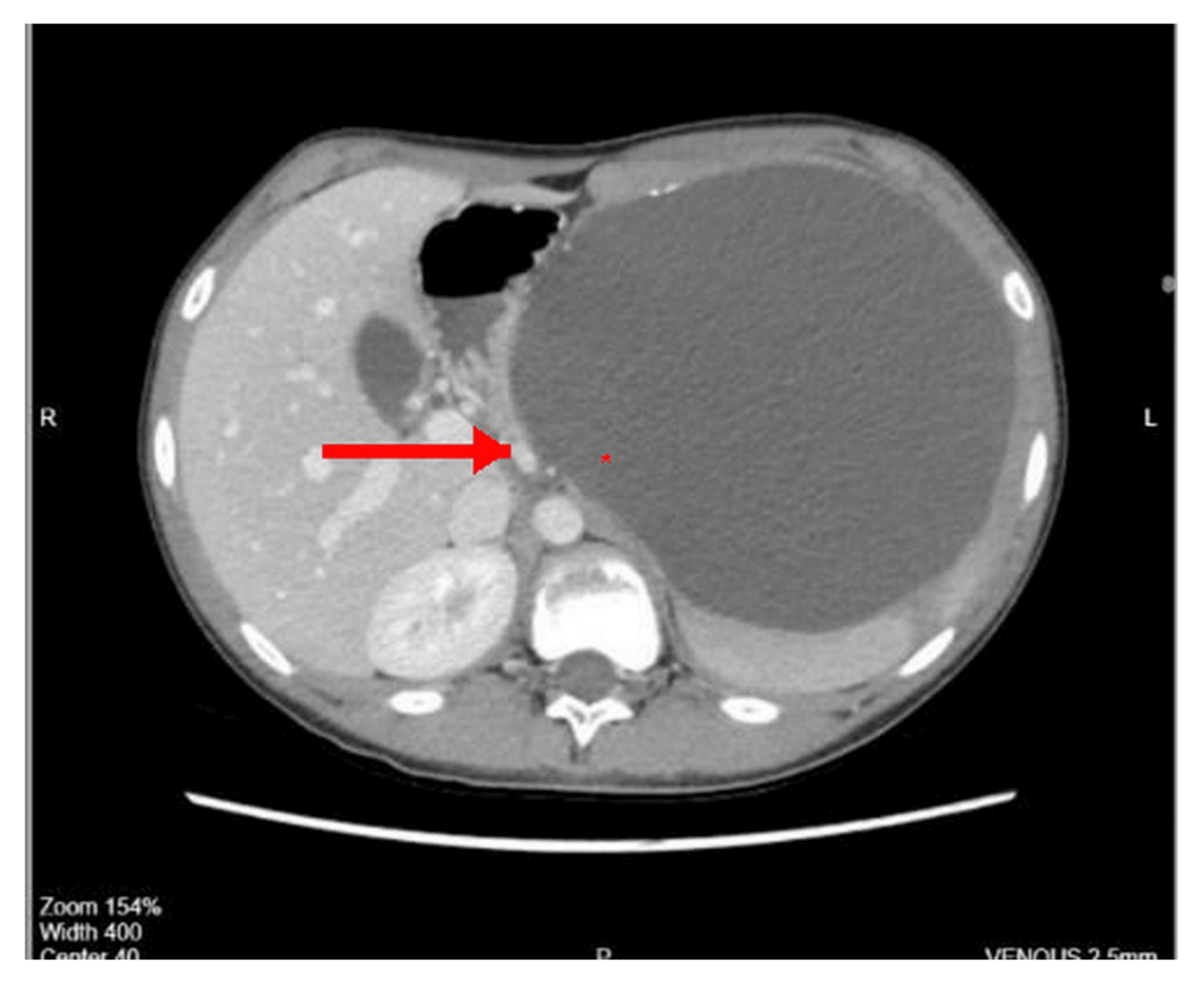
Axial CT scan showing a giant splenic cyst with internal echoes and displacement of adjacent structures. Coronal contrast-enhanced CT image demonstrating a large, well-defined, thin-walled splenic cyst occupying the superior pole with compression of surrounding abdominal organs (asterisk marks cyst cavity).
